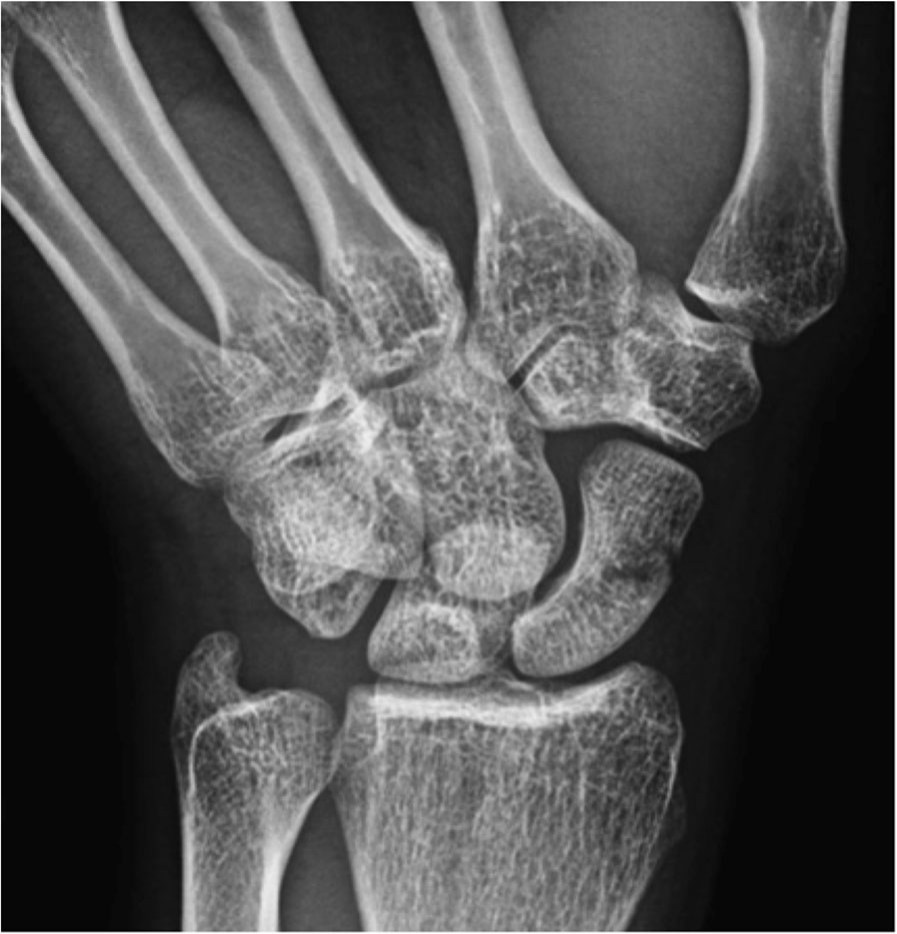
Scaphoid view radiograph of the left wrist in a gymnast with continued radial wrist pain demonstrating grade III scaphoid waist stress fracture
